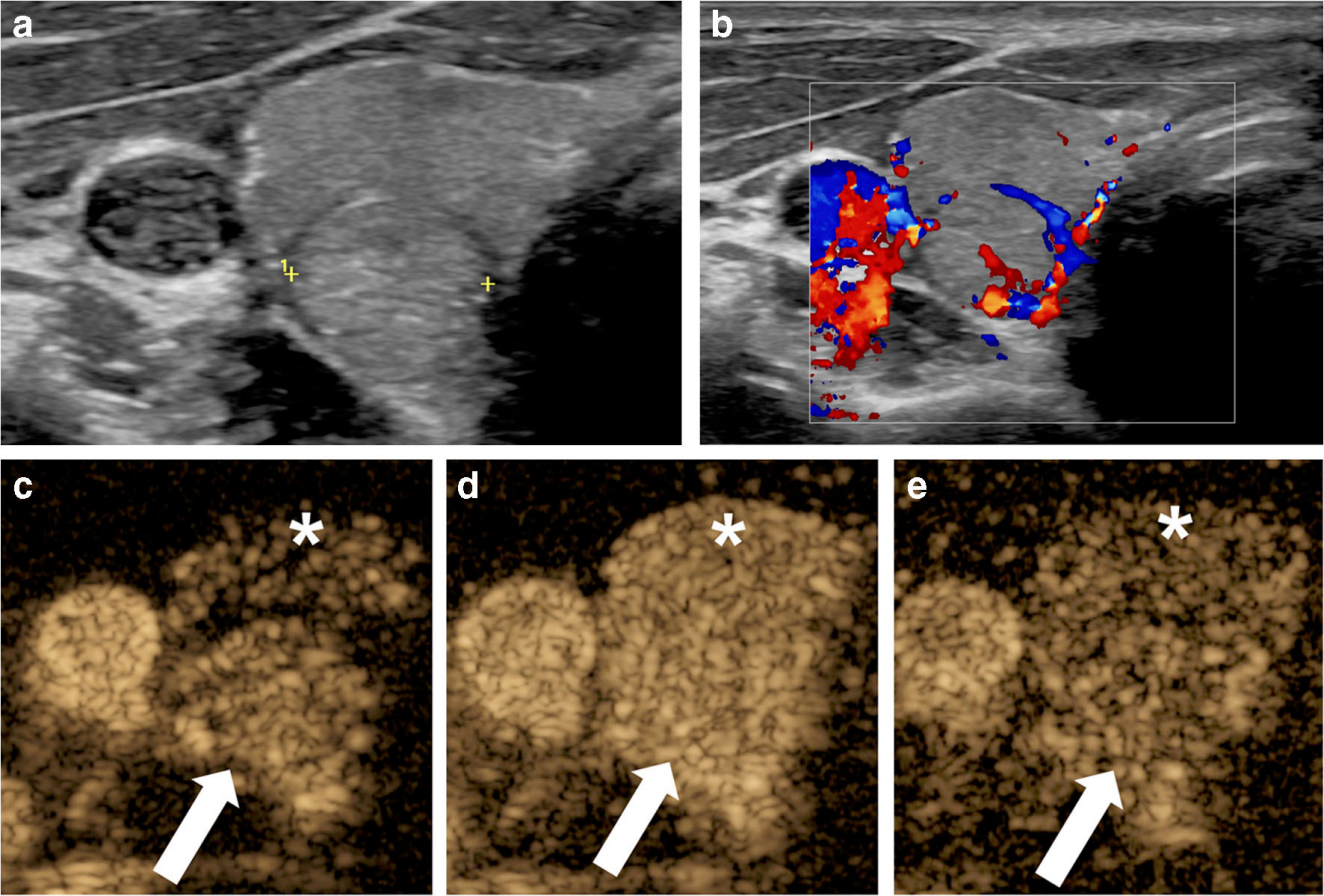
Right thyroid nodule that was incidentally detected on a CT scan of the cervical spine in a 17-year-old boy. The CT was obtained following a motor vehicle collision. a Transverse gray-scale ultrasound (US) of the right thyroid shows a well-circumscribed, solid, iso- to slightly hyperechoic nodule in the lower pole (*calipers*). b Transverse color Doppler US of the right thyroid nodule demonstrates predominantly peripheral vascularity. c–e Transverse contrast-enhanced ultrasound (CEUS) of the right thyroid lobe. Contrast-only mode. There is homogeneous enhancement of the nodule (*arrow*), which is slightly hyperenhancing to the overlying thyroid parenchyma (*asterisk*) in the arterial phase (c) and iso-enhancing in the parenchymal (d) and delayed (e) phases. Overall this is a benign CEUS enhancement pattern. The diagnosis following biopsy was a benign follicular adenoma, oncocytic type (Hurthle cell adenoma)
